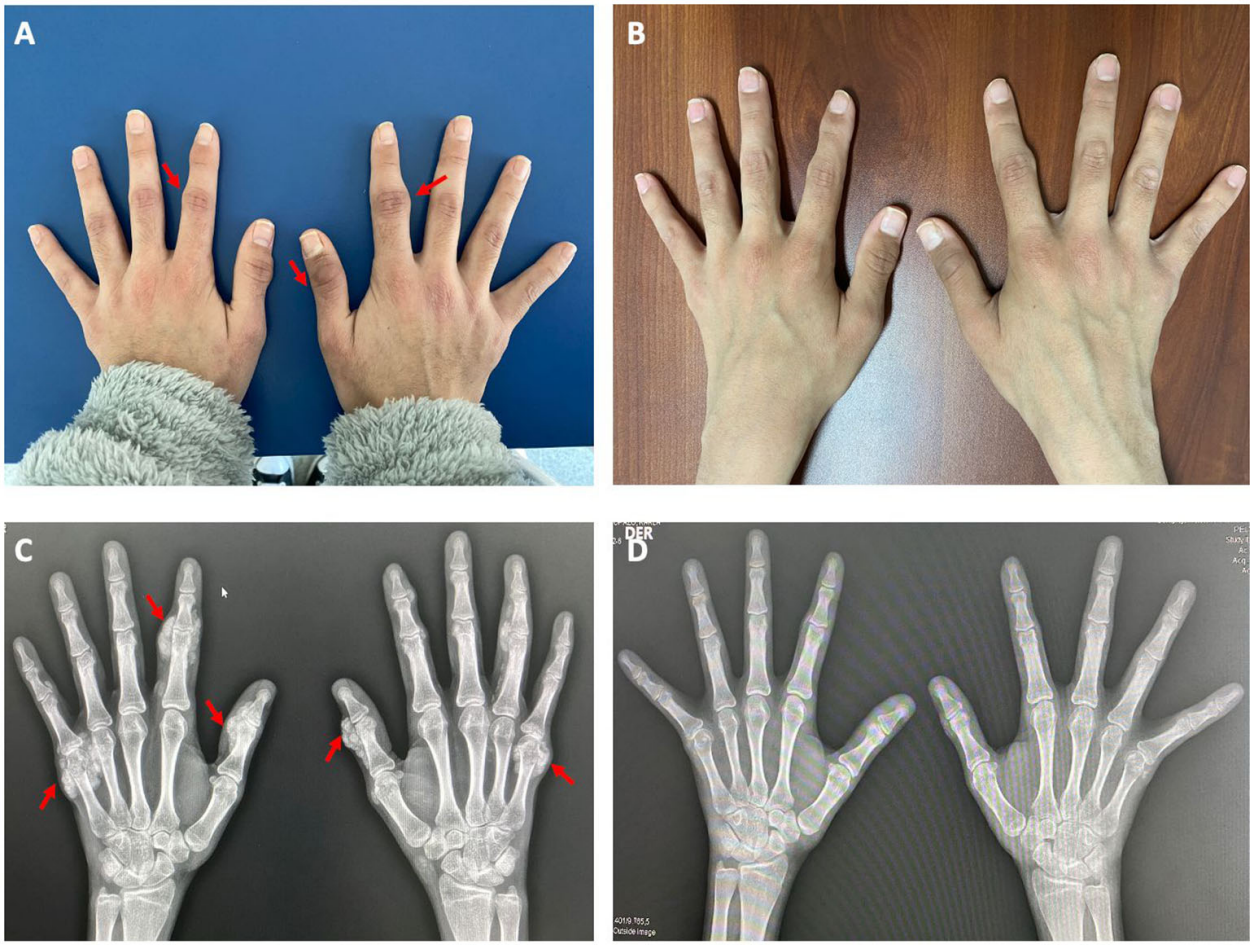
Extraosseous calcifications (amorphous and multilobulated periarticular calcifications) at baseline and 3 months after treatment. (A) and (C) show the photograph of extraosseous calcifications in fingers and hands and the X-ray at baseline, respectively. (B) and (D) show the photograph of both hands and the X-ray without extraosseous calcifications, 3 months after the start of treatment.
